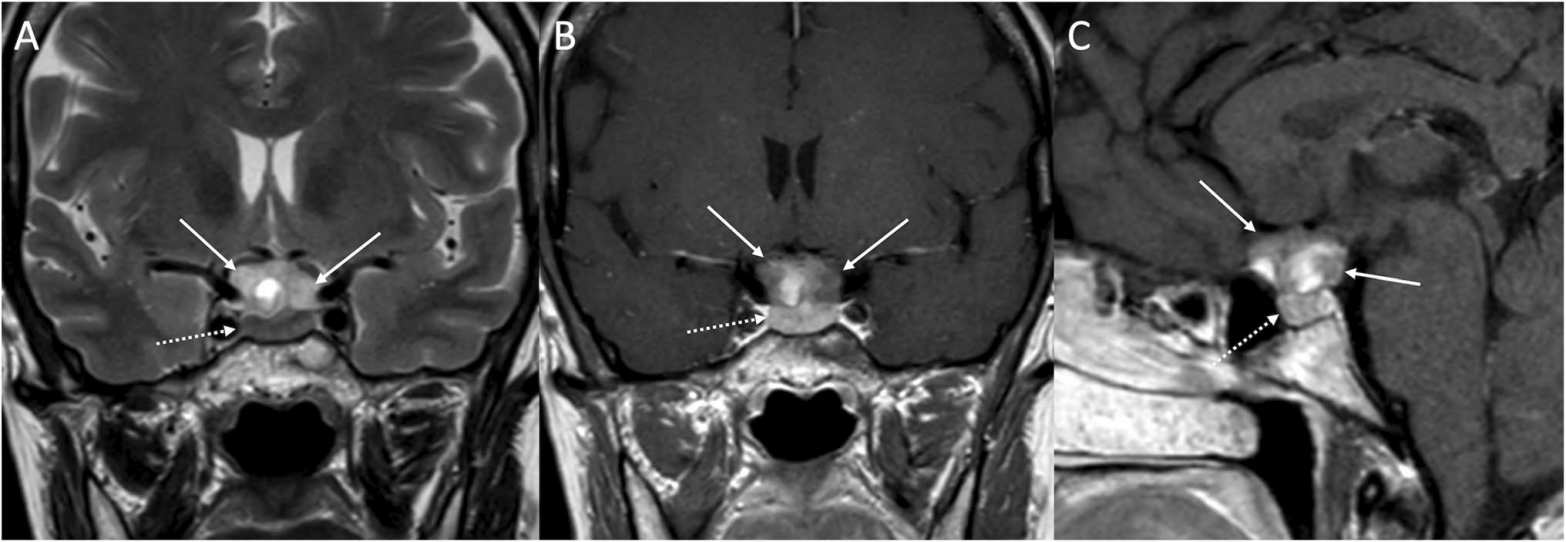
Suprasellar adenoma. Coronal T2- (A), contrast-enhanced coronal (B), and sagittal (C) T1-weighted images show a suprasellar lesion (arrows) dissociable from the underlying pituitary gland (dotted arrows)
